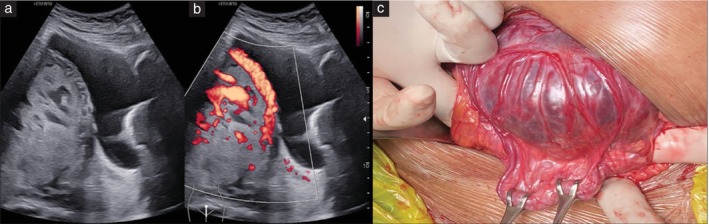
(a) Grayscale ultrasound image in a patient with two previous Cesarean deliveries and anterior placenta previa, showing signs of extensive lower uterine segment remodeling (placental bulge, myometrial thinning and loss of clear zone). (b) On color Doppler imaging, features of uteroplacental vascular remodeling (subplacental hypervascularity, lacunae and lacunae feeding vessels) and serosal hypervascularity (bridging vessels and bladder wall interruption) were observed. (c) The intraoperative image shows a thinned lower uterine segment with a placental bulge, with massive vascularity on the serosal surface running craniocaudally, with anastomosis to the vesical vessels. A diagnosis of high‐grade placenta accreta spectrum was made based on the intraoperative appearance and the failure of placental separation.
